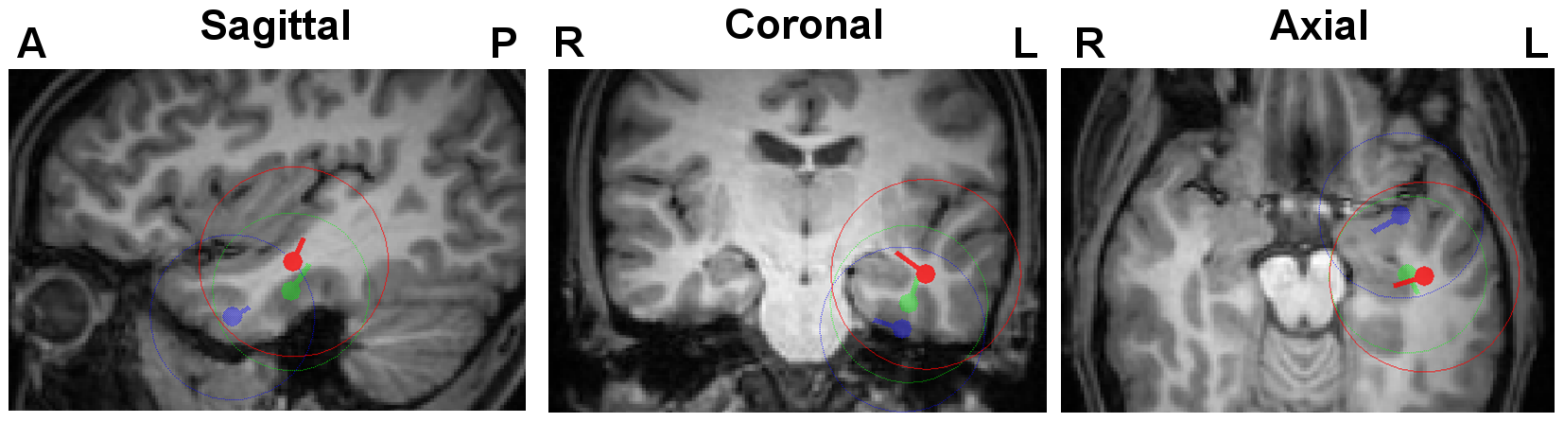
Differences of EEG, MEG and combined EEG/MEG localizations. FT9 centroids and spread spheres plotted on T1w-MRI for combined EEG/MEG (red), MEG (green) and EEG (blue) using head model *6C_Cal*. The centroid location of the combined reconstruction was used for the selection of MRI slices and all results were projected on these slices.
